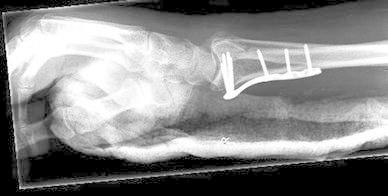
Lateral X-ray showing a slightly prominent distal edge of the plate
